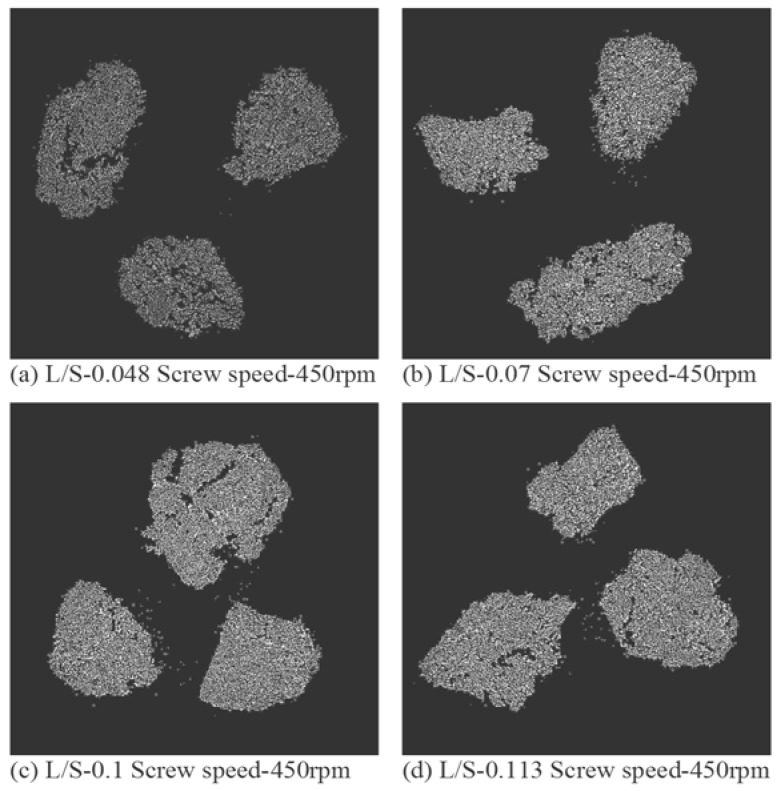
X-ray tomographic images of granules produced using Pearlitol 50C at varying L/S (screw speed, 450 rpm).
